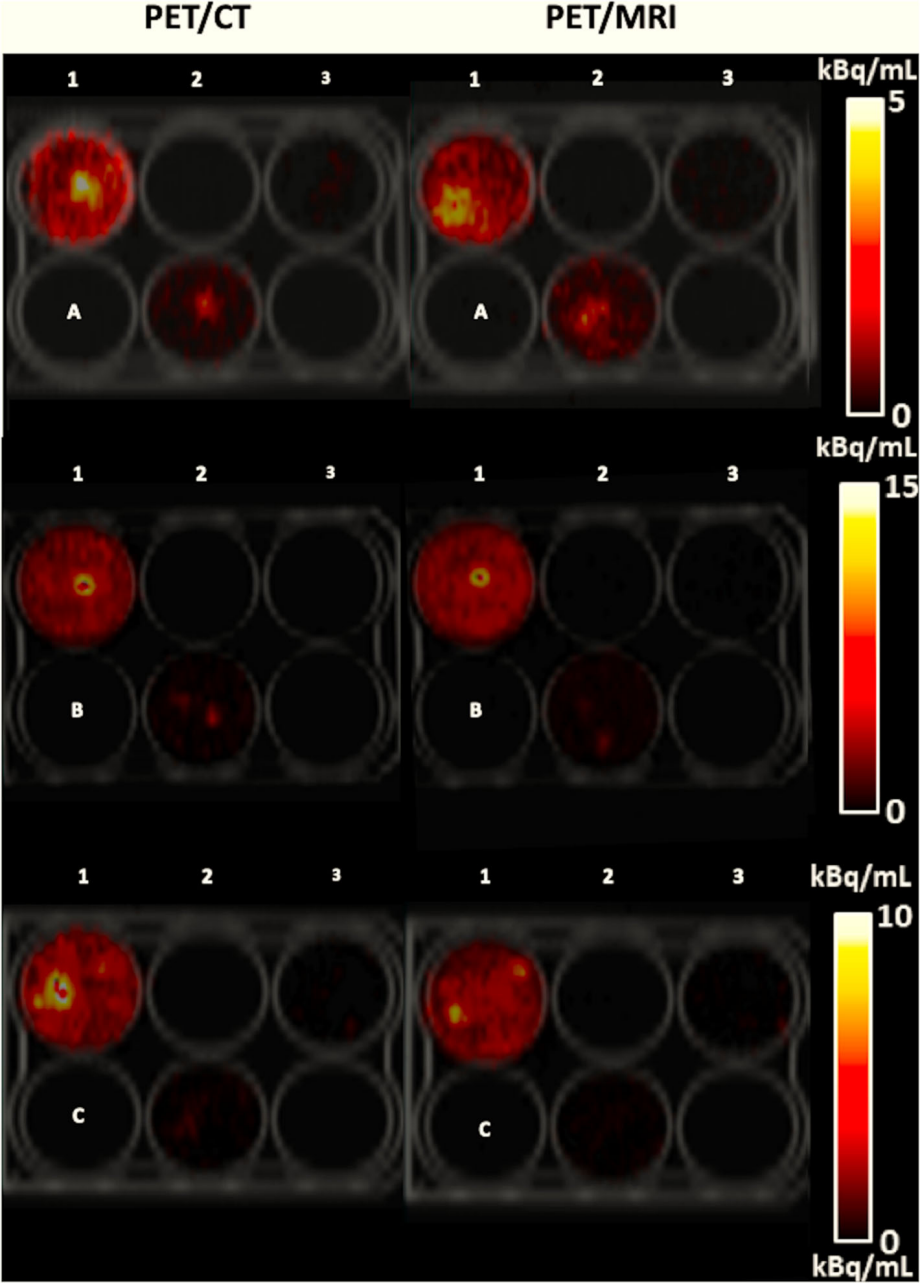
PET images of 6-well plates containing ^89^Zr-labeled Jurkat cells acquired using PET/CT and PET/MRI together with their corresponding co-registered CT image. Cell numbers and specific activities per well (kBq/10^6^ cells; indicated parenthetically) were as follows: (A1) 1.36 × 10^6^ cells (2.70). (A2) 5.20 × 10^5^ cells (2.18). (A3) 1.45 × 10^5^ cells (1.57). (B1) 3.83 × 10^5^ cells (31.91). (B2) 6.75 × 10^4^ cells (27.77). (B3) 1.00 × 10^4^ cells (18.37). (C1) 8.45 × 10^5^ cells (7.83). (C2) 1.65 × 10^5^ cells (3.57). (C3) 6.10 × 10^4^ cells (6.93)
